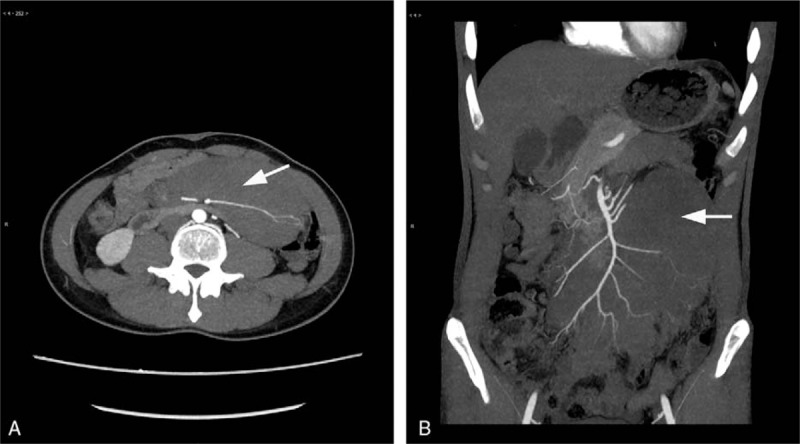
Preoperative computed tomography (CT) shows tumor extension. Representative images of transverse section (A) and coronal section (B). The arrow indicated the lesion. CT = computed tomography.
